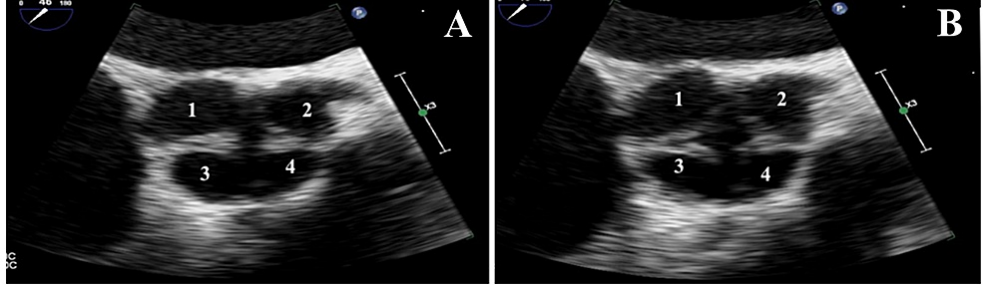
Transesophageal echocardiogram taken four days after admission showing quadricuspid valve (1-4) during isovolumetric contraction phase (A) and early systolic phase (B)
